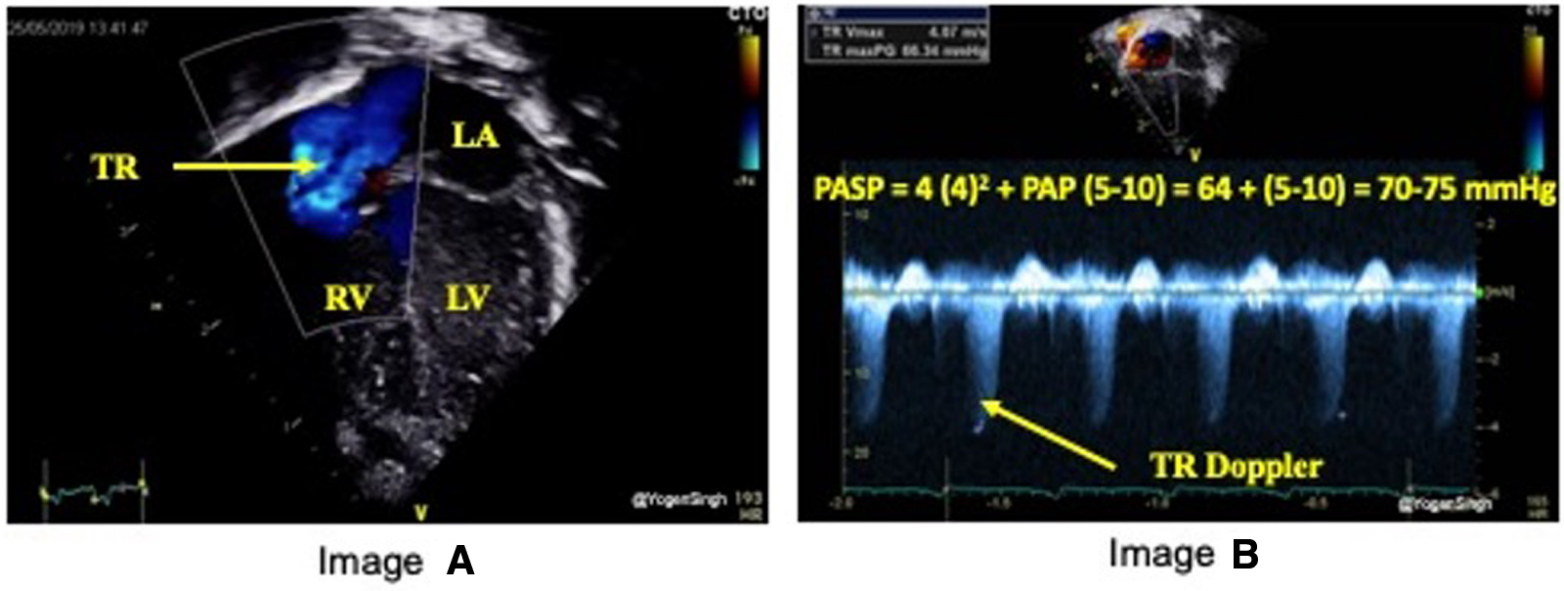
Estimation of pulmonary artery systolic pressure (PASP). Image “A” shows TR jet in apical 4 chamber view (A4C) and image “B” with Doppler assessment of tricuspid regurgitation (TR) – in this case a gradient of around 64 mmHg (TR jet velocity of 4 m/s) between right ventricle (RV) and right atrium (RA) suggesting PASP between 70 and 75 mmHg (64 + RA pressure of 5–10 mmHg).
